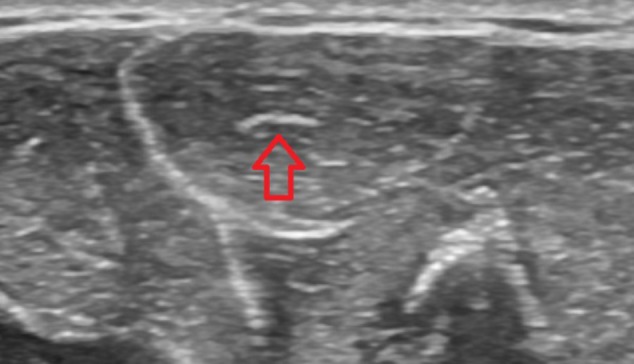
Ultrasound imaging of spindle-shaped PL with a visible tendon inside of the muscle’s belly (red arrow).
